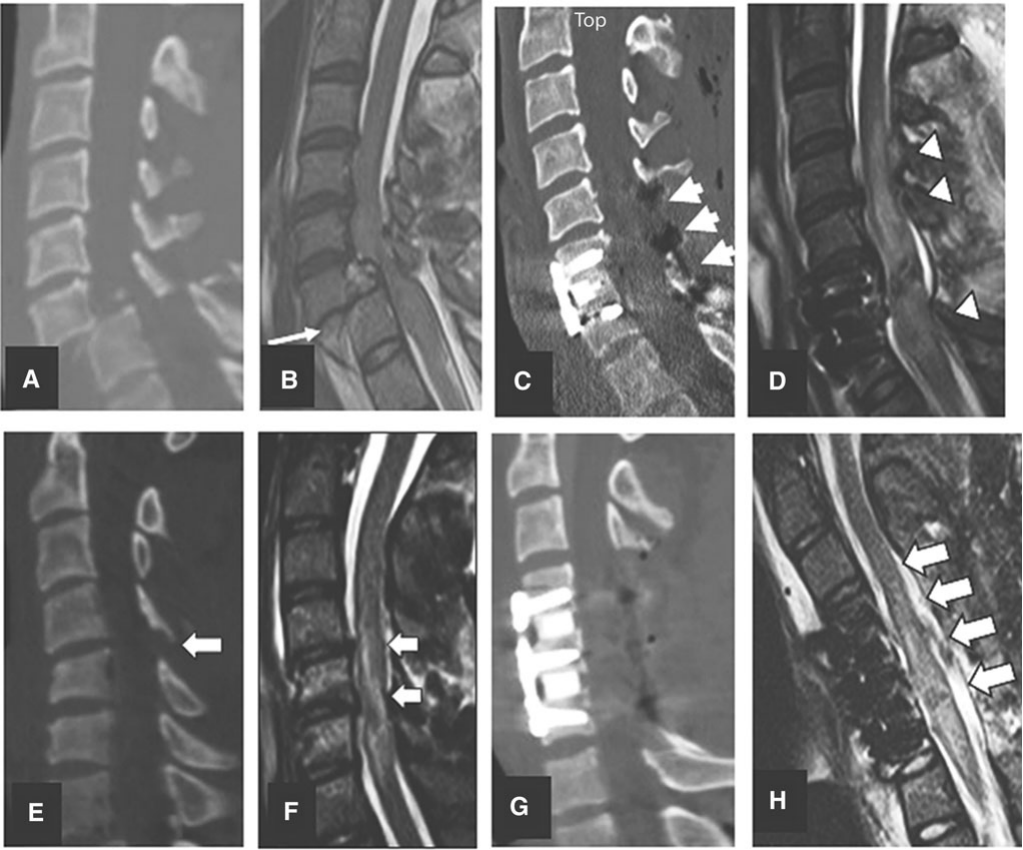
Midsagittal CT and MRI images belonging to two different patients managed by anterior cervical discectomy and fusion (ACDF) and laminectomy. Upper row indicates a C6/C7 translation rotation injury (A and B) with evidence of spinal cord compression. (C) and (D) show one level ACDF and two levels of laminectomy with inadequate decompression of the spinal cord at C4 and C5 and C7. (E-H) indicate a flexion compression injury and evidence of spinal cord compression at C5 and C6 segments. The patient had ACDF at C5/6 and C6/7 with three levels of laminectomy and posterior spinal fusion (G and H) with adequate decompression of the spinal cord (arrows).
